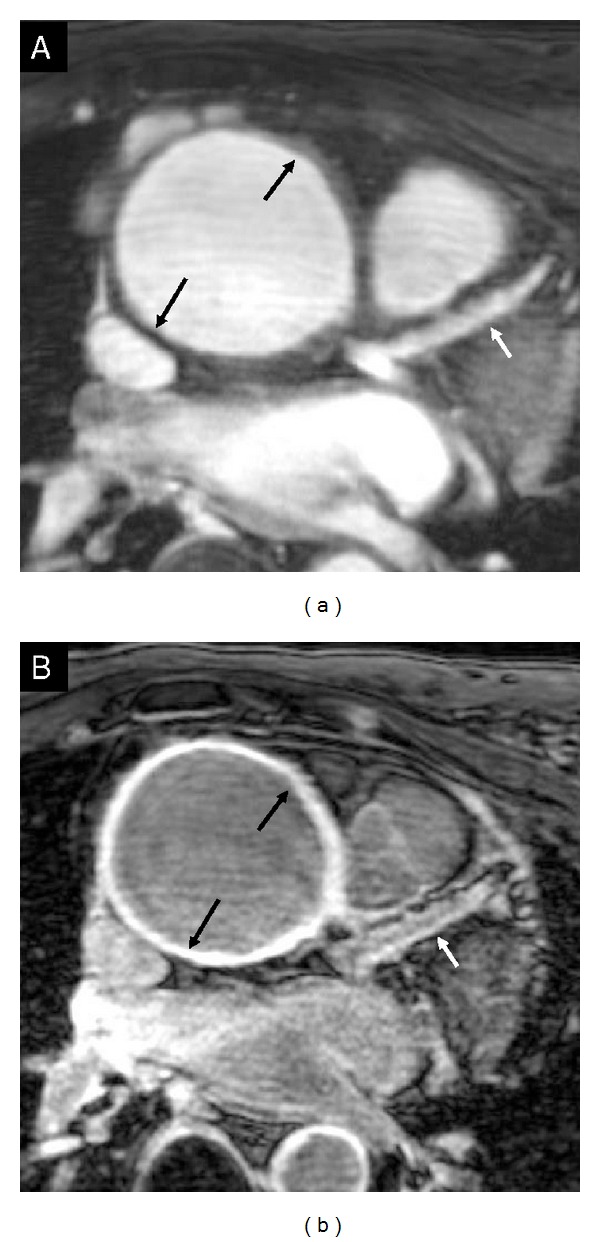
Cross-sectional view of the ascending aorta (black arrows) and longitudinal view of the proximal part of the left anterior descending coronary artery (white arrows) with T2Prep (a) and postgadolinium enhancement MR imaging (b). There is strong vessel wall enhancement after administration of contrast on both the aorta and the coronary artery.
